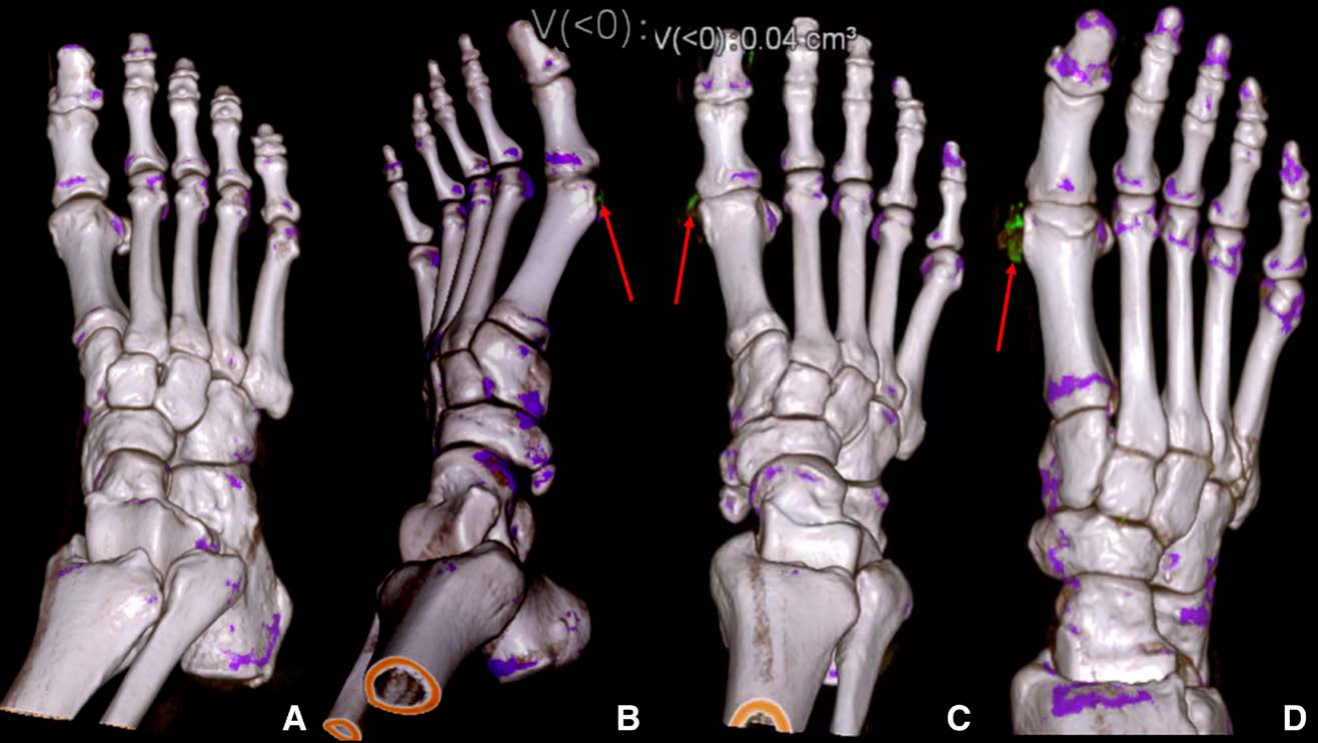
3D reconstruction DECT images of the first metatarsophalangeal joints with urate indicated as green (as shown by the red arrows). (a), score of 0; (b), score of 1; (c), score of 2; (d), score of 3. DECT = dual-energy computed tomography.
